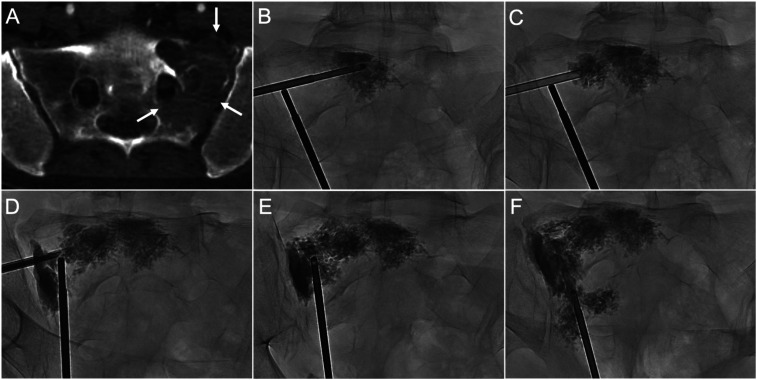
Application of side-opening injection cannulae in the treatment of complex sacral lesions. (A) Axial CT image demonstrates extensive osteolytic disruption of the left S1 foraminal, sacroiliac joint, and anterior sacral margins (*arrows*). (B–D) PA radiographs demonstrate sequential cement injections via the transiliac approach from the sacral body to the ipsilateral ala. (E–F) AP radiographs after long-axis sequential injections filled the remaining areas in the sacral ala to achieve a contiguous distribution. The side-opening was directed away from the foramen during cement injections. No foraminal, sacroiliac joint or extraosseous leakage was demonstrated.
